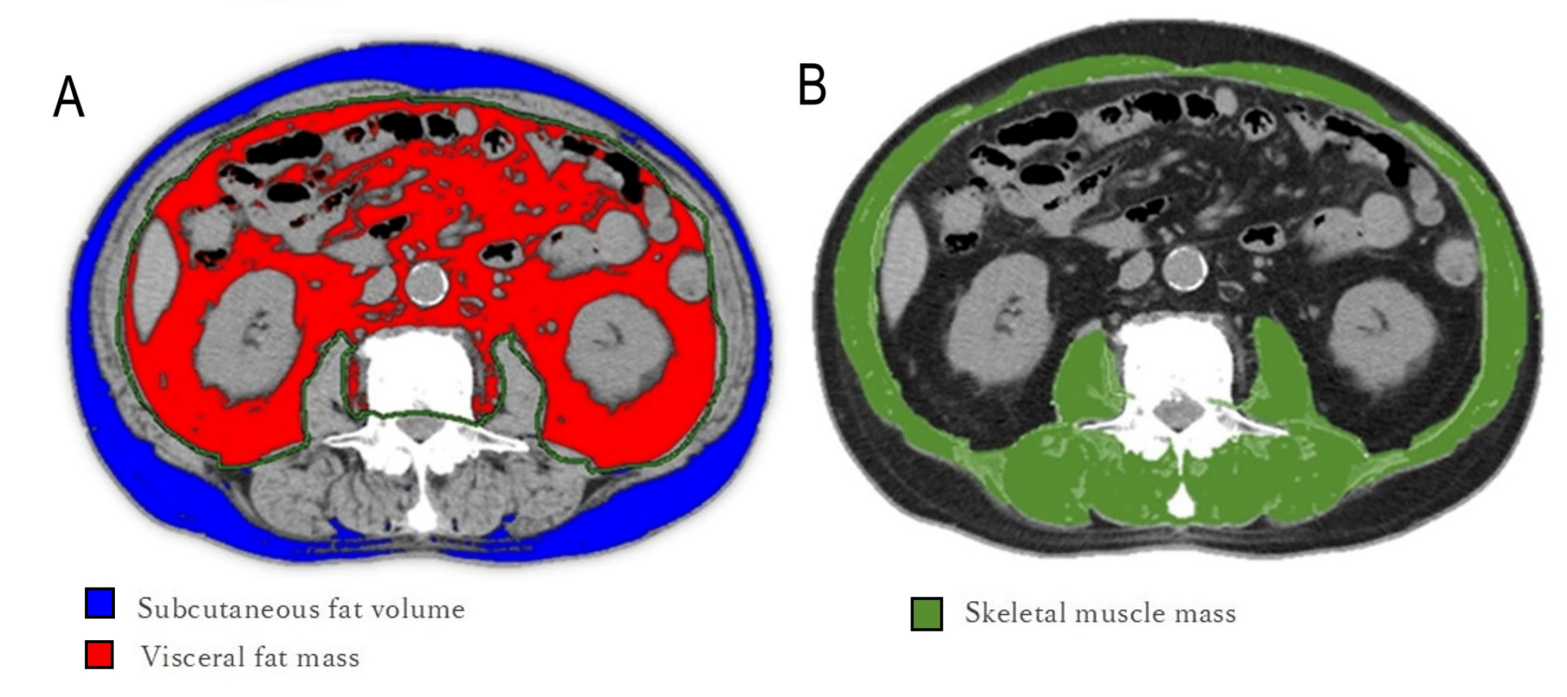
Measuring body composition using computed tomography (A) The subcutaneous and visceral fat contents were measured using L3 level images; the Hounsfield Unit (HU) value segmentation of fat was set to -150 to -70 HU. (B) The skeletal muscle mass was traced as the region of interest for all skeletal muscles at the L3 level; the cross-sectional area of the skeletal muscle at the L3 level was measured using CT value-based segmentation of -29 and 150 HU.
